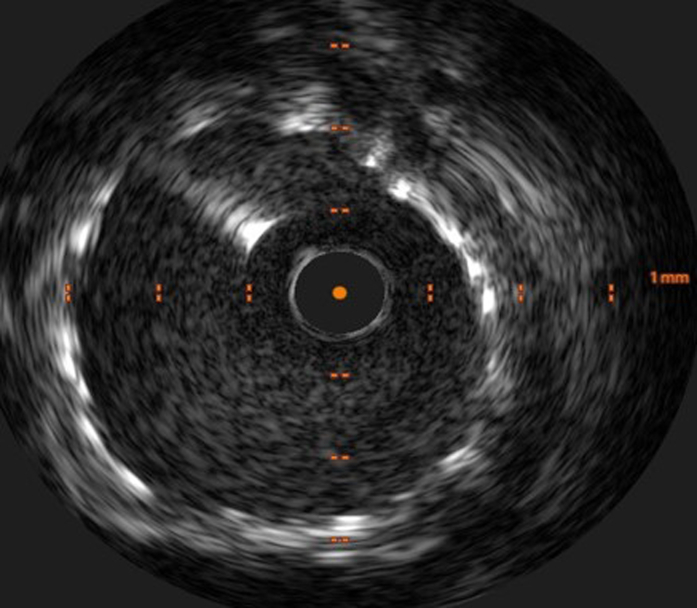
Intravascular Ultrasound After Percutaneous Coronary Intervention to the Right Coronary Artery
